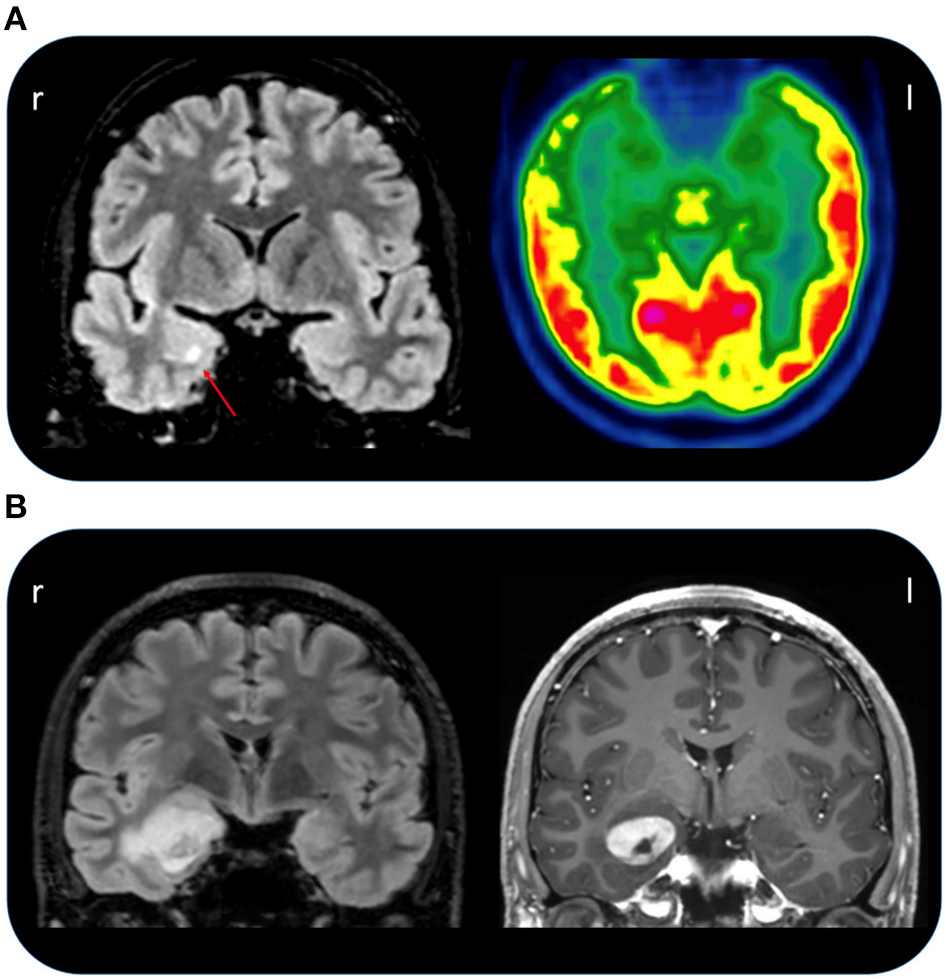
(A) Coronal FLAIR MRI of patient 2 (left) showing a hyperintense right amygdala with red arrow pointing to an area of increased signal intensity (red arrow). On the right, the FDG-PET image of the same patient showing right temporal hypometabolism involving the temporal pole and mesial regions. (B) Coronal FLAIR MRI of patient 4 (on the left) showing a temporo-mesial lesion that on T1 imaging (on the right) present a clear gadolinium enhancement suggesting a tumoral origin.
